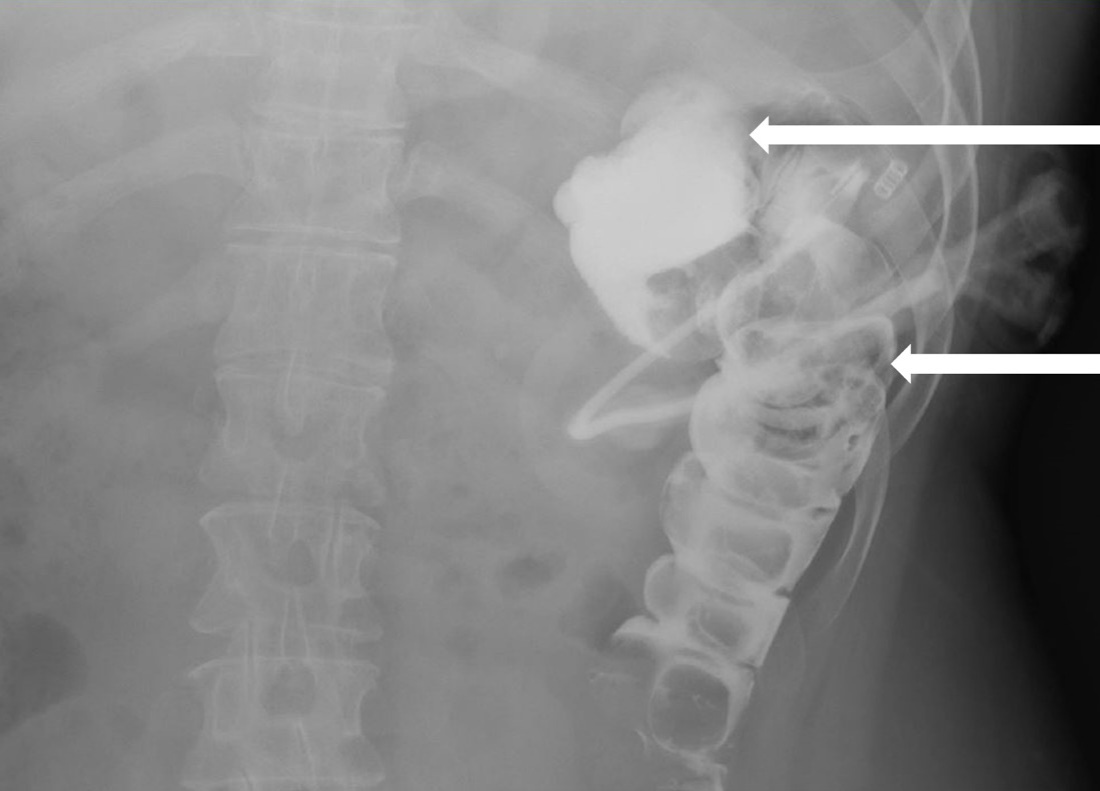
Gastrografin radiography showing percutaneous endoscopic gastrostomy tube in the colon with contrast filling colon at the splenic flexure (top arrow) and proximal descending colon (bottom arrow) suggestive of colocutaneous fistula formation.
